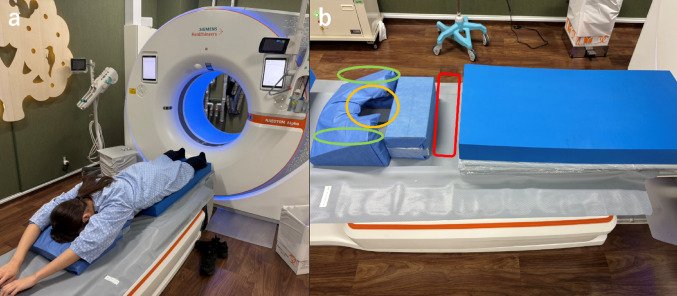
Prone positioning protocol for CT acquisition. a Patient positioned prone on the CT table using the standardized support setup. b Support arrangement and intended positioning. The face is placed within the head support (yellow circle), the arms are positioned on the arm supports (green circles), and the breasts are allowed to hang freely in a dependent, non-compressive configuration in the designated opening (red rectangle) to minimize deformation
